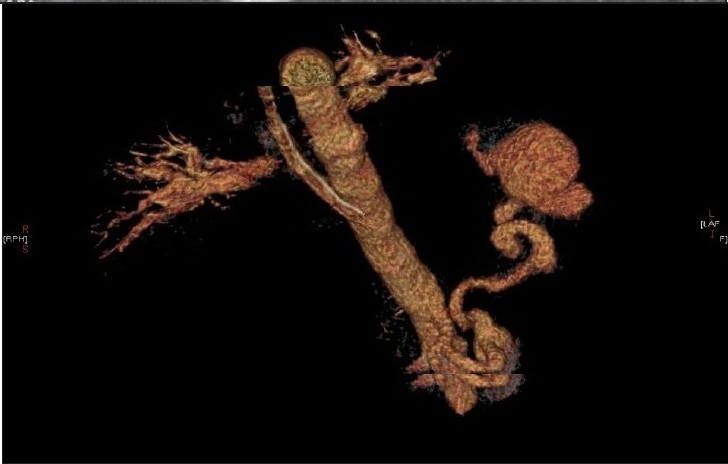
Reconstruction view of the spiral CT scan shows the splenic artery aneurysm. There is no evidence of calcification in the wall of the splenic aneurysm
